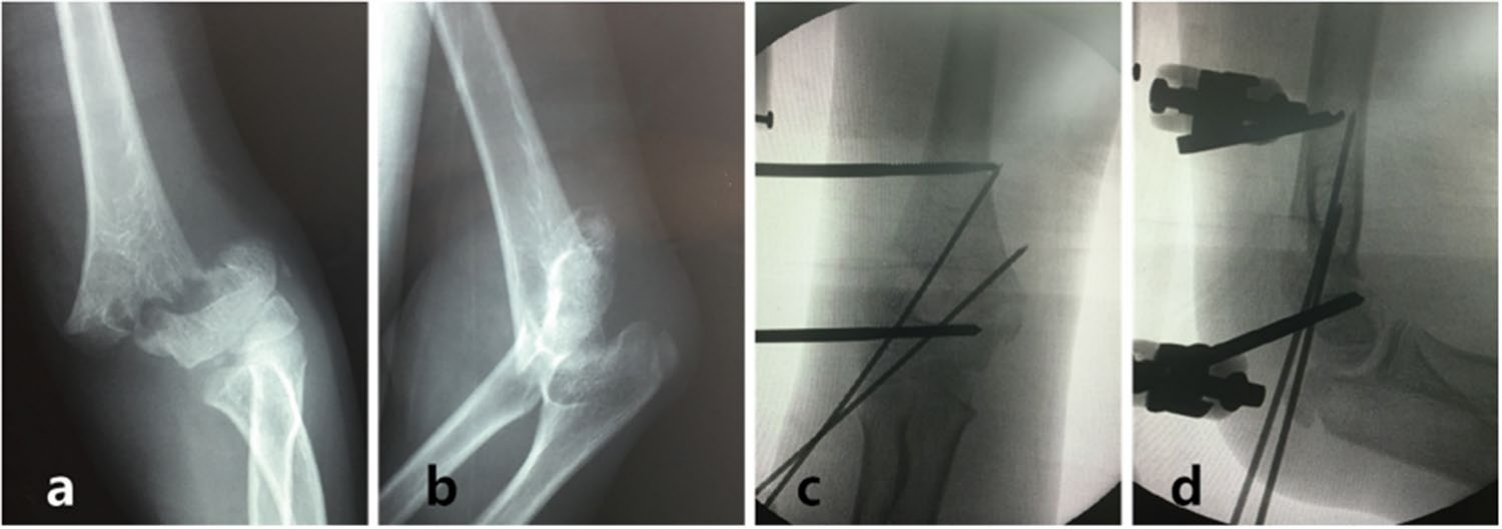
A 13-year-old boy diagnosed with flexion-type SHF of his left elbow. (a, b) The anteroposterior (AP) and lateral radiographic images on admission; (c, d) The post-operative AP and lateral radiographs.
